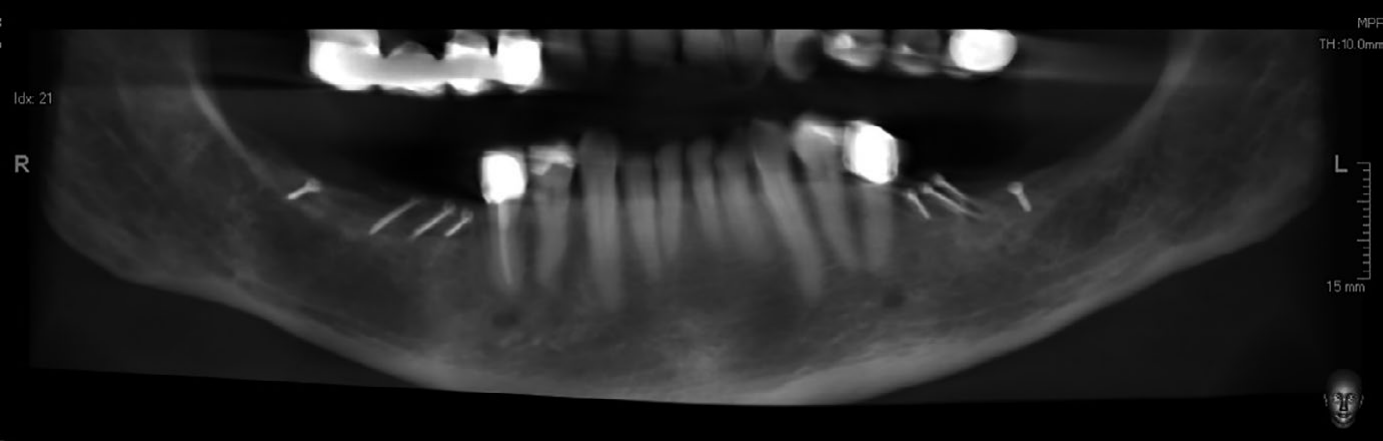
CBCT before implantation: significant vertical bone gain after 4 months of healing in both quadrants
